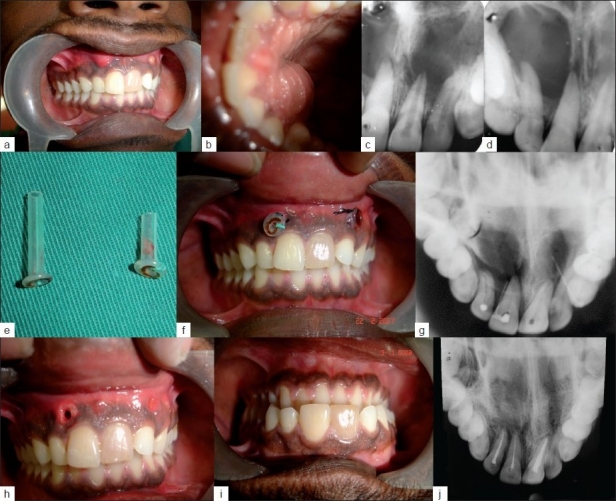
a) Picture showing swelling in labial vestibule region, b) Picture showing swelling in palatal mucosa, c) Radiograph of 11, 12 regions which reveal radiolucency involving periapical region, d) Radiograph of 21, 22 regions which reveal radiolucency involving periapical region, e) Picture of the cannula, f) Insertion and stabilization of cannula by interrupted sutures, g) Occlusal radiograph confirming the position of cannula, h) Picture of the clinical appearance of the channel, i) Picture of the healed aperture, j) Occlusal radiograph of 11, 12 and 21 region showing trabecular pattern throughout lesion after 1year
